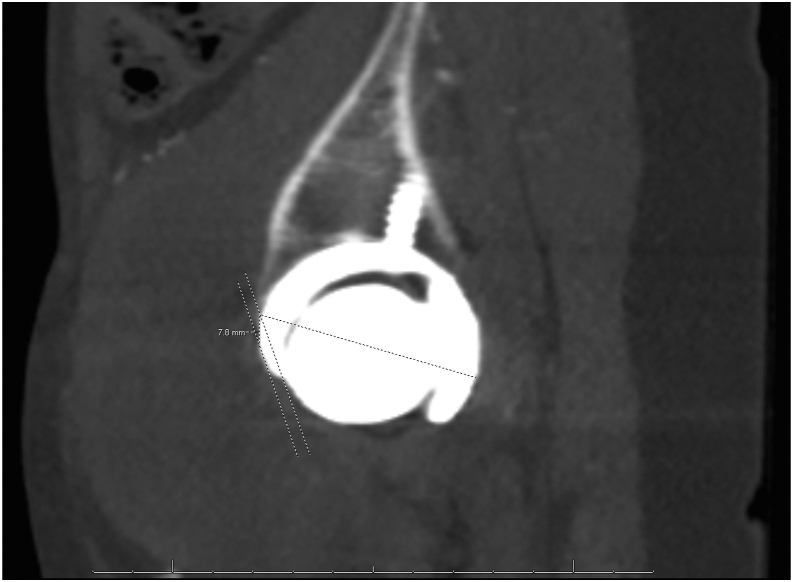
Sagittal view of the right hip. CT scan demonstrating 7.8 mm of anterior acetabular component overhang.
